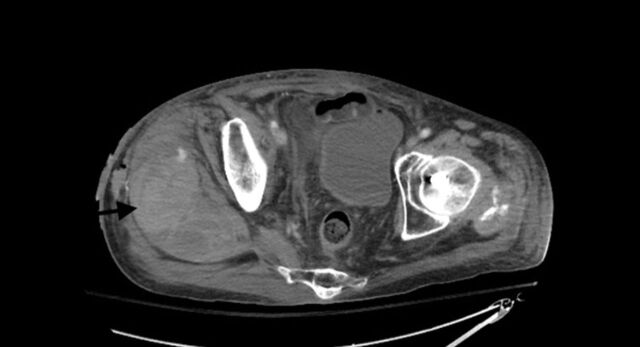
Computed tomography with contrast of the pelvis shows a 9 × 8 × 12-cm right-sided hematoma (arrow).
